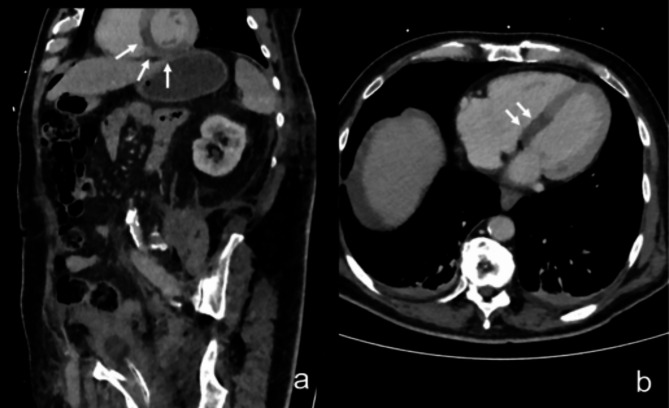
Incidental finding of myocardial hypoperfusion on venous phase abdominal CT imaging for evaluation of postoperative hemorrhage after radical prostatecomty. In this image, reconstructed abdominal CT images in oblique (a) and axial (b) view are shown. Imaging was conducted due to suspicion of abdominal bleeding after previous radical prostatectomy. Myocardial hypoperfusion located at the basal and midventricular level of the septum and the inferior wall can be delineated (white arrows, images a and b).
